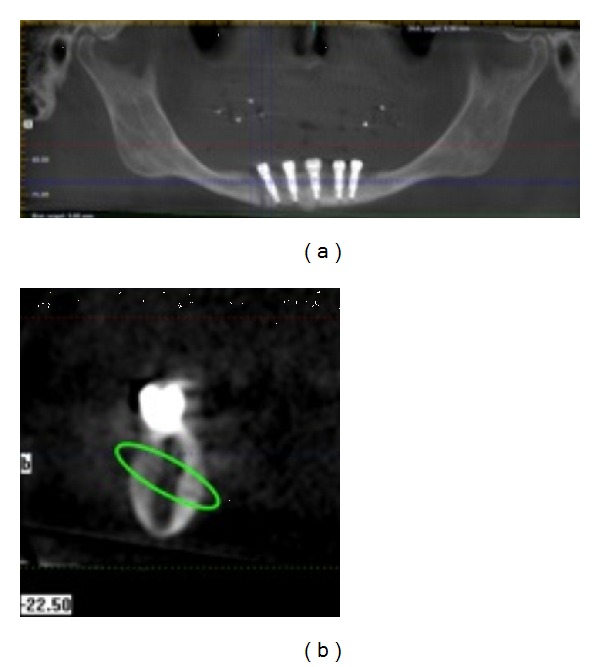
The CBCT (a) and a slice (b) where the hairline fracture is evident.
